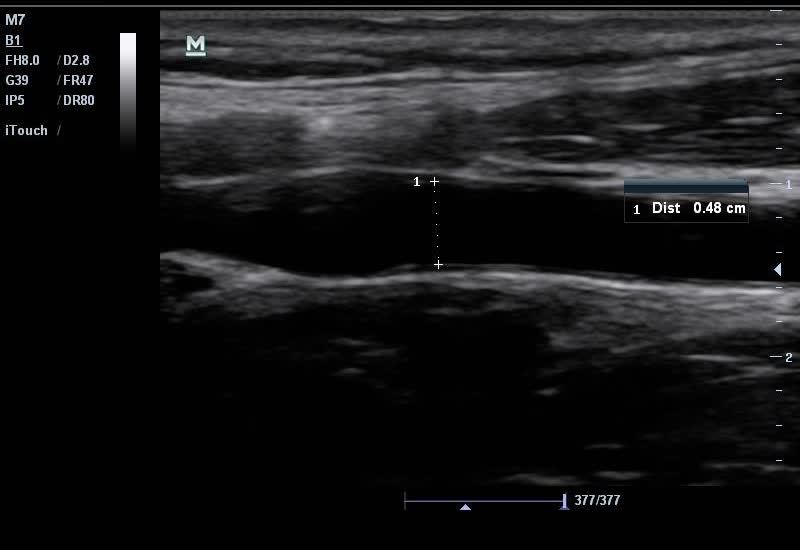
Ultrasound image of common carotid diameter measurement obtained within 0.5 cm of the carotid bulb and measured intima to intima.
